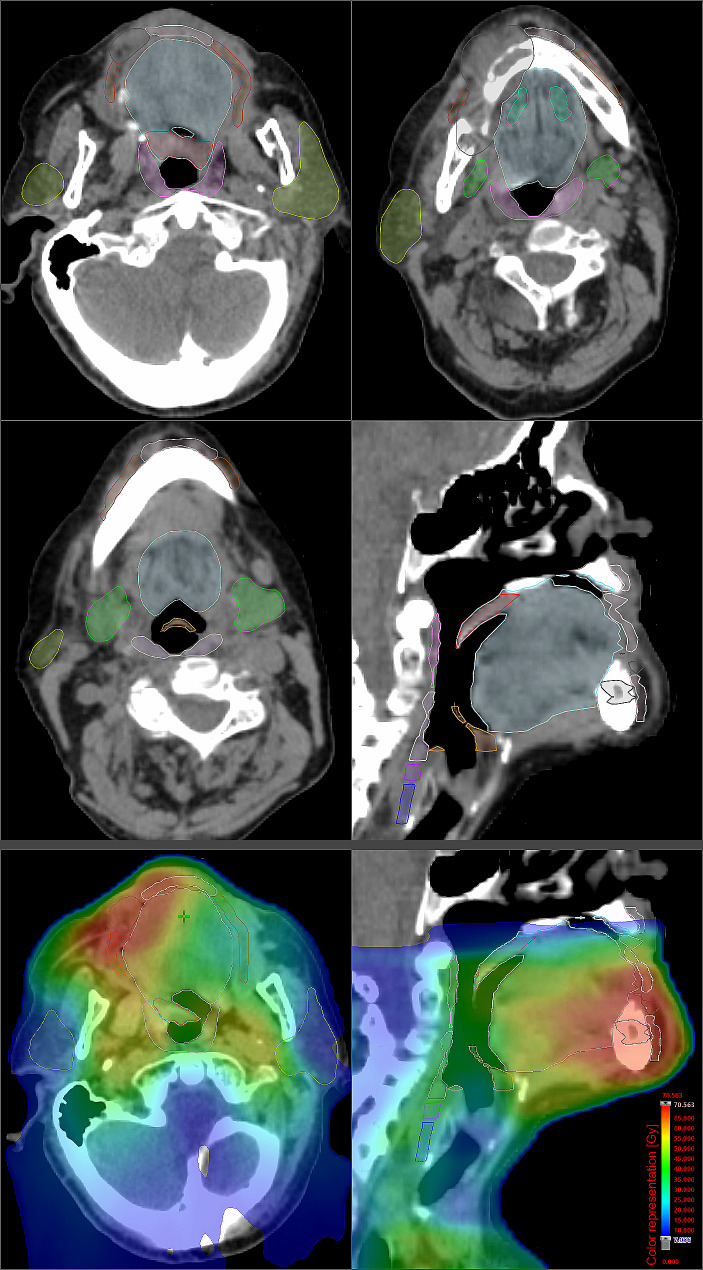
Organs at risks analyzed regarding chronic xerostomia, dysgeusia and dysphagia in older adults with head and neck squamous cell carcinoma undergoing definitive radiotherapy. Top: Axial and sagittal views of a radiation treatment planning CT, showing the contoured organs at risk: left and right parotid glands (chartreuse), left and right submandibular glands (green), left sublingual gland (turquoise), salivary glands of the left and right buccal mucosa (brown), salivary glands of the labial mucosa (pale pink), soft palate (red), extended oral cavity (light blue), supraglottic larynx (orange), superior pharyngeal constrictors (pink), middle pharyngeal constrictors (lilac), inferior pharyngeal constrictors (violet), cricopharyngeal muscles (dark violet). The gross tumor volume is shown in dark blue. Bottom: Color representation of the dose distribution in axial and sagittal sections of the aforementioned radiation treatment planning CT.
